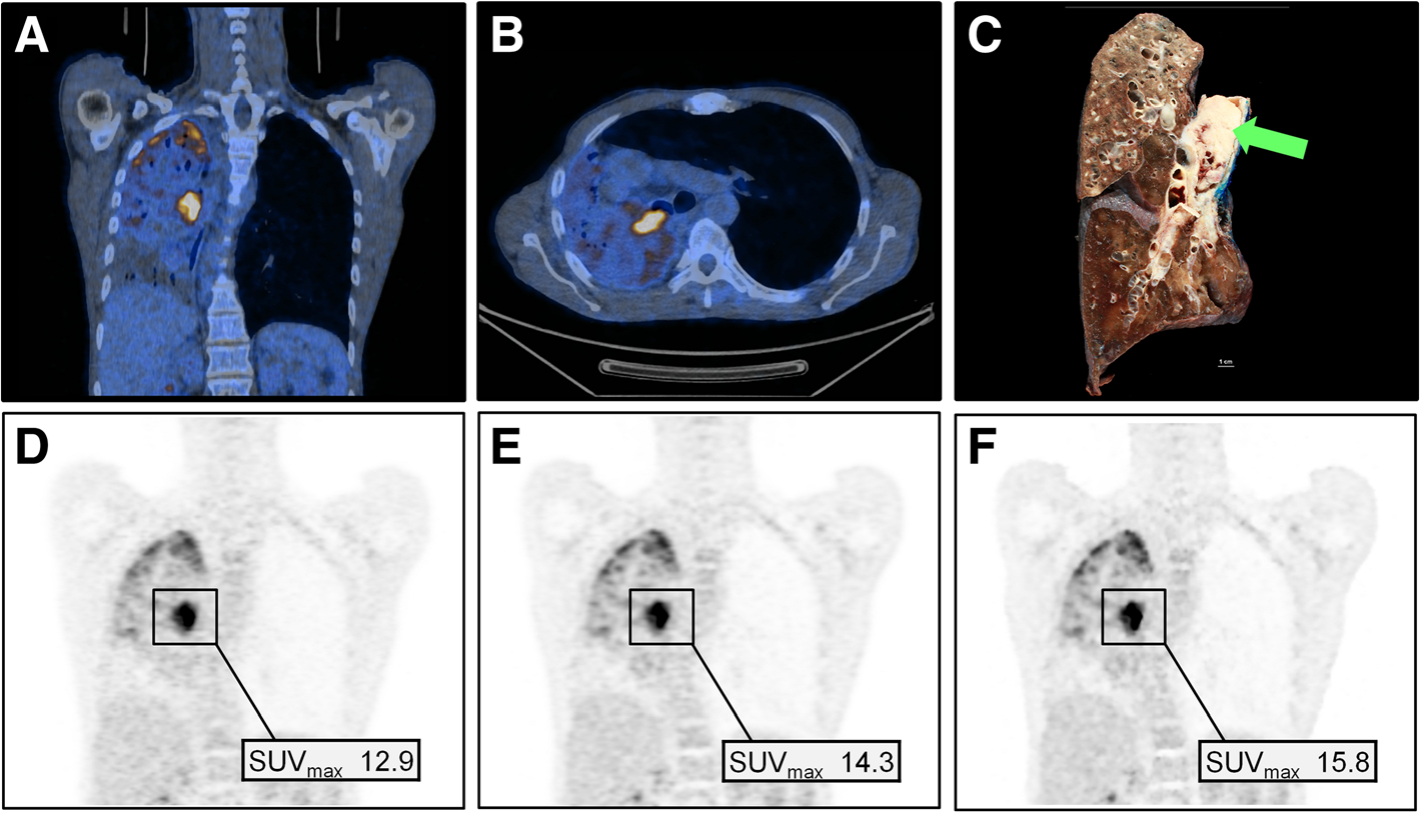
Representative images of a 53-year-old man with a body mass index of 19.1 kg/m^2^ and 66 kg body weight who underwent 18F-FDG PET/CT for staging of lung cancer. The patient was injected with 99.8 MBq of 18F-FDG (i.e., 1.5 MBq/kg body weight). Coronal and axial co-registered PET/CT images (a, b) show a highly 18F-FDG-avid tumor invading the right main bronchus, which was confirmed as squamous cell carcinoma (arrow) after right-sided pneumonectomy (c). Coronal PET images show OSEM_TOF_ (d), OSEM_PSF_ (e), and BSREM_600_ (f) reconstruction together with SUV_max_ of the primary tumor
